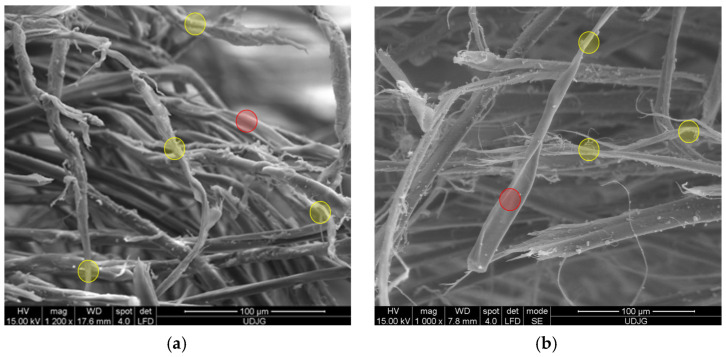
Fiber deformation: yellow circles—necking, red circles—flattening: (a) Twaron^®^ CT 709 with PVB matrix, 32 layers, after a hit with a 9 mm FMJ bullet [70], (b) front view of layer 1, from a panel of 15 layers of Twaron^®^ T730, hit by a projectile .357 SIG, v_0_ = 448 m/s.
